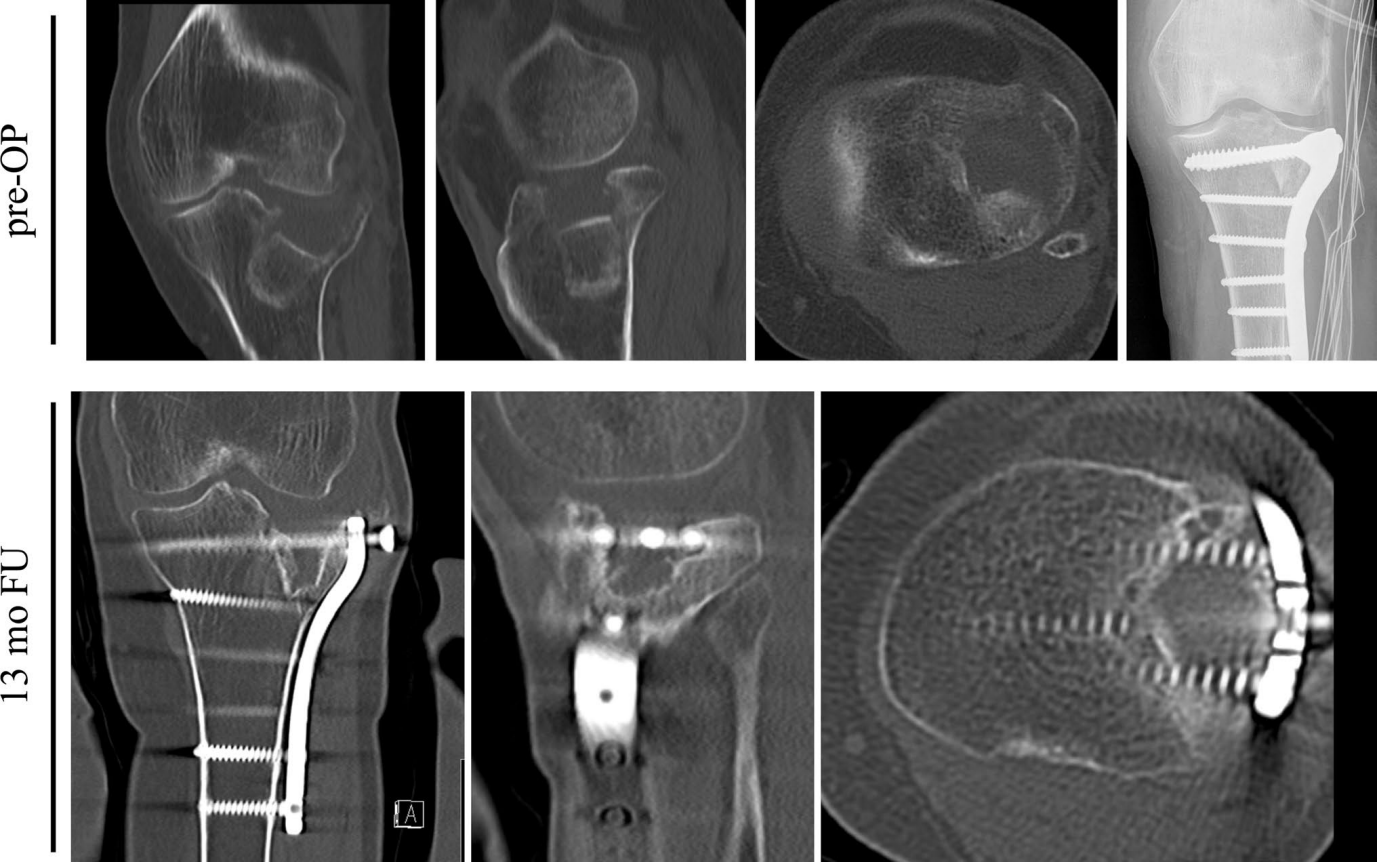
A 78-year-old female with a lateral depression fracture (AO 41-type B2, involving antero-latero-lateral, antero-latero-central segments, *pre-OP*) after a trip and fall. Surgical treatment included an anatomic reduction, transplantation with an autogenic cortico-cancellous iliac crest graft, and fixation with an anterolateral plate. A computed tomography scan at the 13-month follow-up revealed a severe osteochondral defect with a complete dissolution of the autogenic graft. The patient demonstrated a severe vitamin D deficiency of 3.4 ng/mL and persistent hypocalcemia
